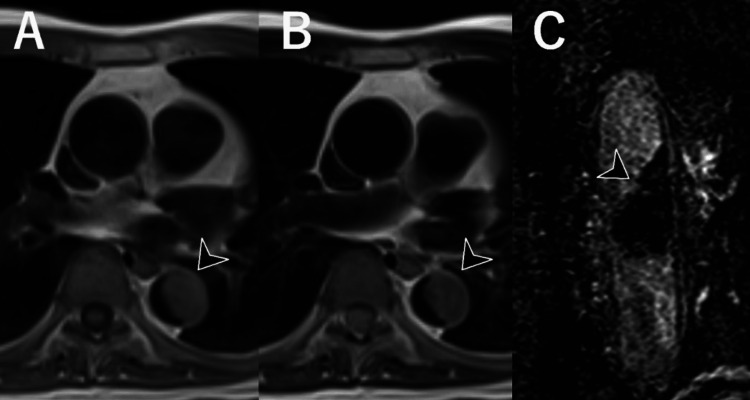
Contrast-enhanced magnetic resonance images. The lesion exhibits mildly high signal intensity on T1-weighted imaging (A). Moreover, a slightly higher signal intensity than that of muscle is noted on T2-weighted imaging (B). No apparent contrast enhancement is observed on subtraction imaging (C).
